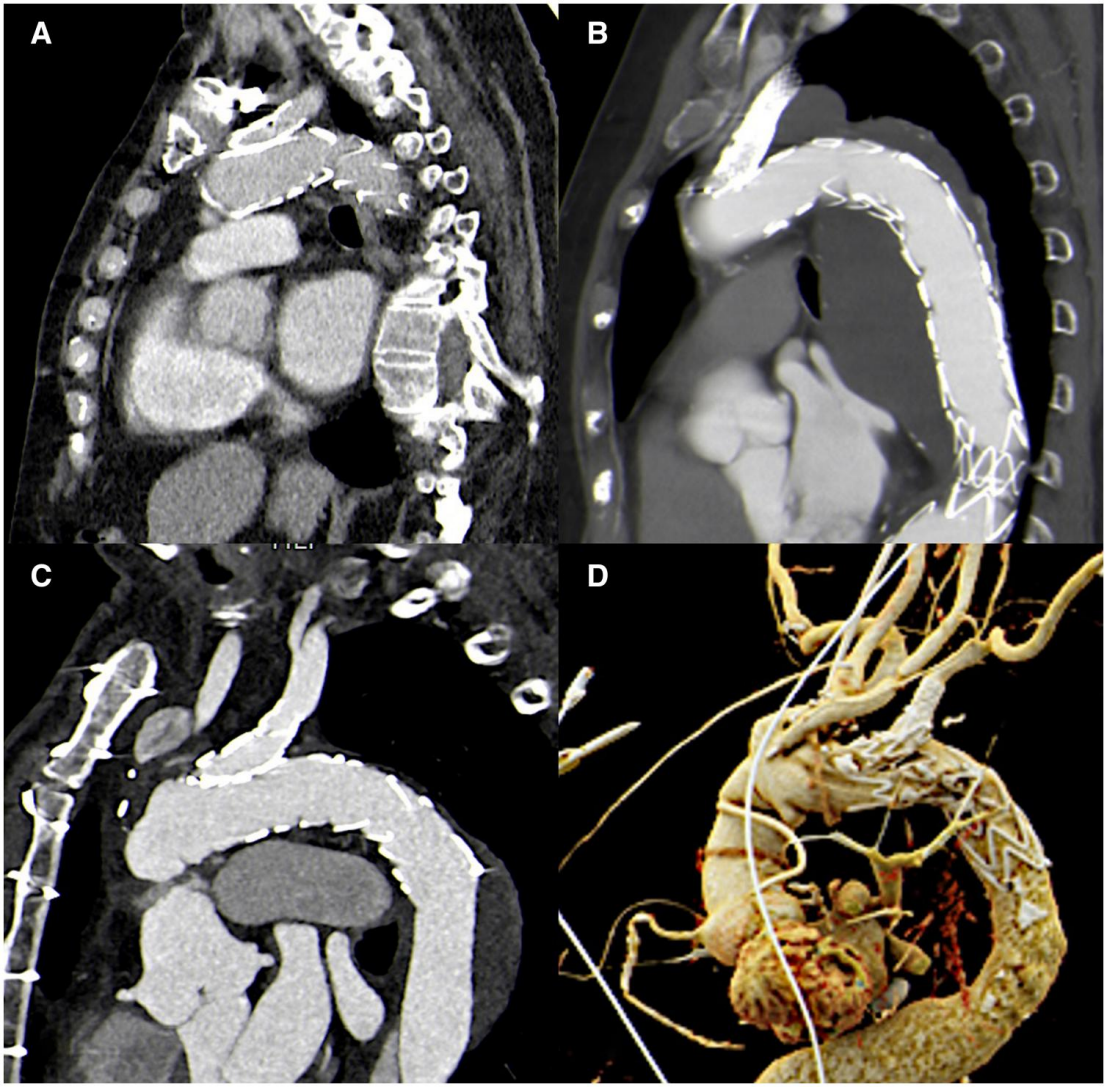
(A–D) Completion CT angiography scans focused on the LSA side branch of all patients; imperfect insertion of the side branch into the LSA can be observed in all cases as well as sealing of the LSA by extension stent grafts.
